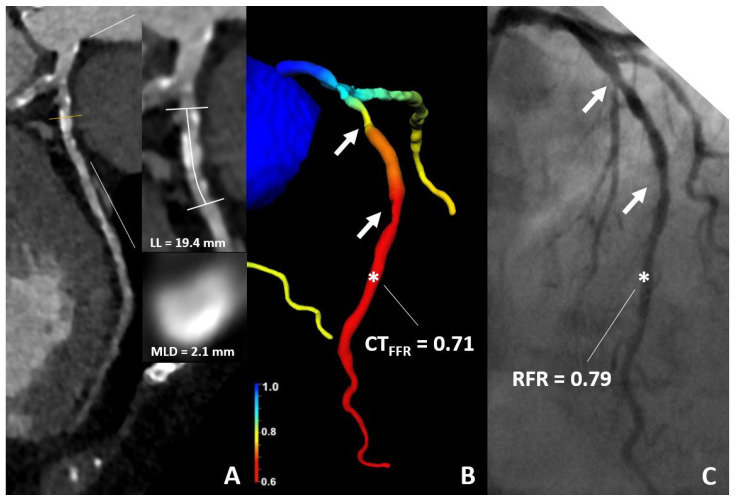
76-year-old woman. (A) CT demonstrates sequential 50% stenoses of the LAD. (B) The CT-FFR value was of 0.71 (star). (C) ICA confirmed ischemia with an RFR-value of 0.79 (star); RFR Cut-Off ≤ 0.89. CT, computed tomography; CT-FFR, coronary computed tomography angiography based fractional flow reserve; RFR, resting full cycle Ratio; LL, lesion length; MLD, minimal luminal diameter.
